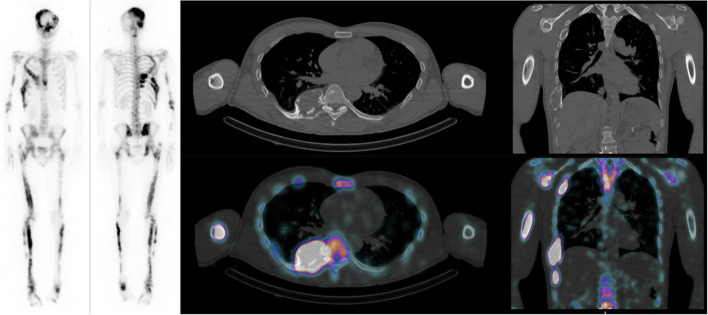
Forty-year-old male patient with Mazabraud syndrome: Mean SUV_max_ and SUV_mean_ of 11 and 6.7 g/mL, respectively. SBS: 46.3, FGF-23: 175 (10–50 pg/mL), CTX: 531 pg/mL, P1NP: 383 (15–78 μg/L), ALP: 277 (36–108) U/L, scoliosis angle: 11 degrees.
